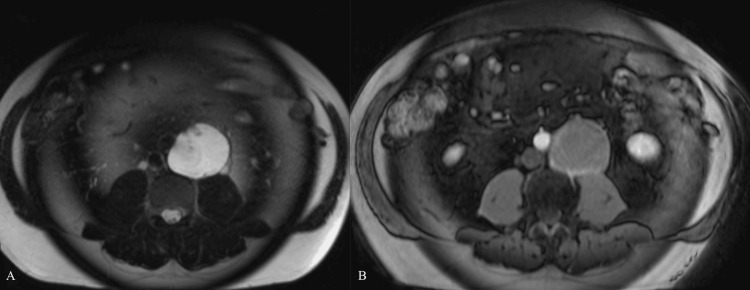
Axial MRI images of the abdomen T2 (A) and T1 post-contrast (B) MRI images of the abdomen demonstrate a well-circumscribed, T2-hyperintense retroperitoneal mass with thin peripheral rim enhancement without internal nodularity or solid enhancement. MRI: Magnetic resonance imaging
